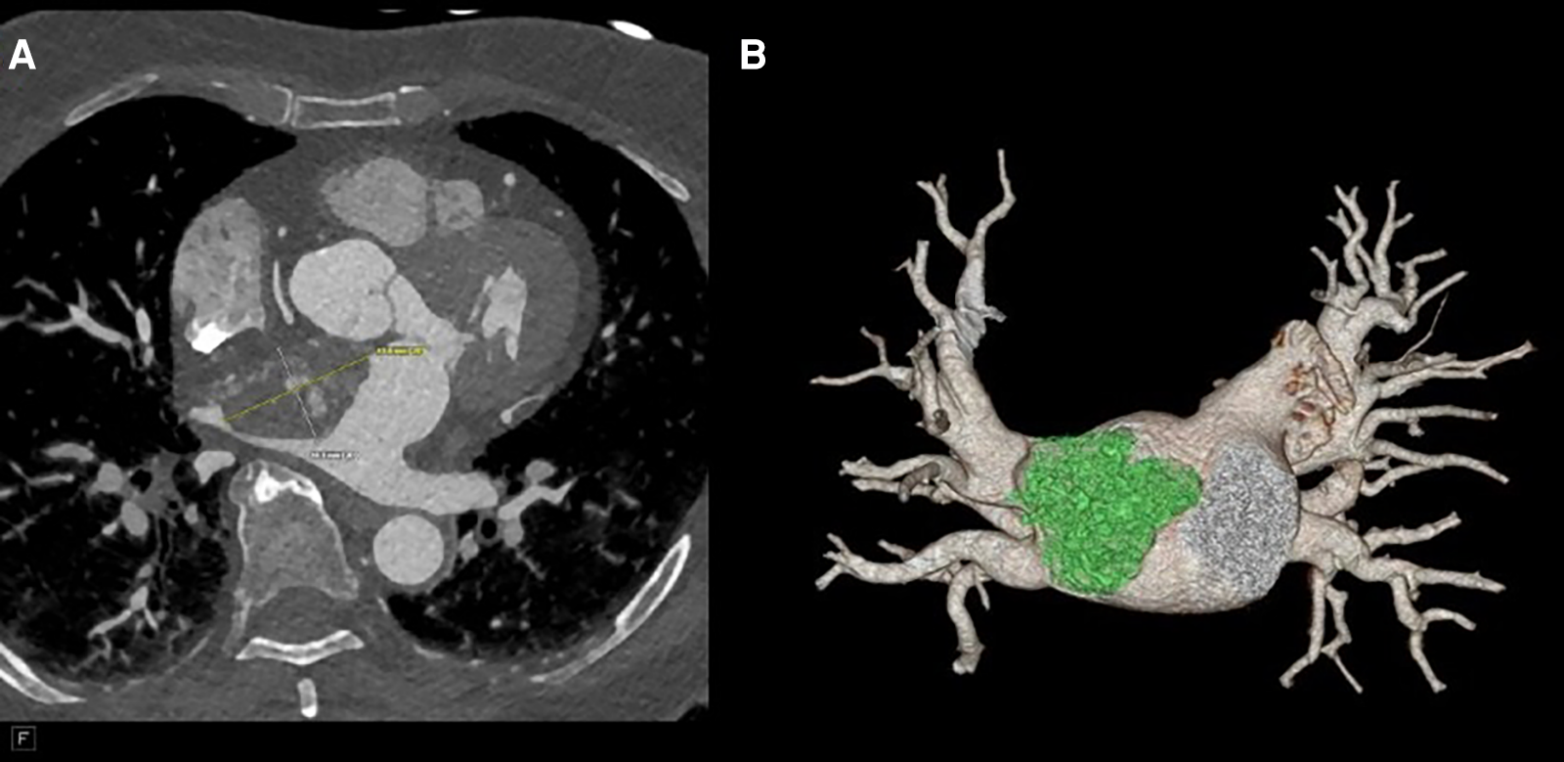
(A) CTA revealing a 41 mm × 27 mm × 33 mm broad-based lobulated mass in the left atrium that extends into the mitral annulus. (B) CTA reconstruction to show the mass extending to the right upper pulmonary vein (RUPV).
